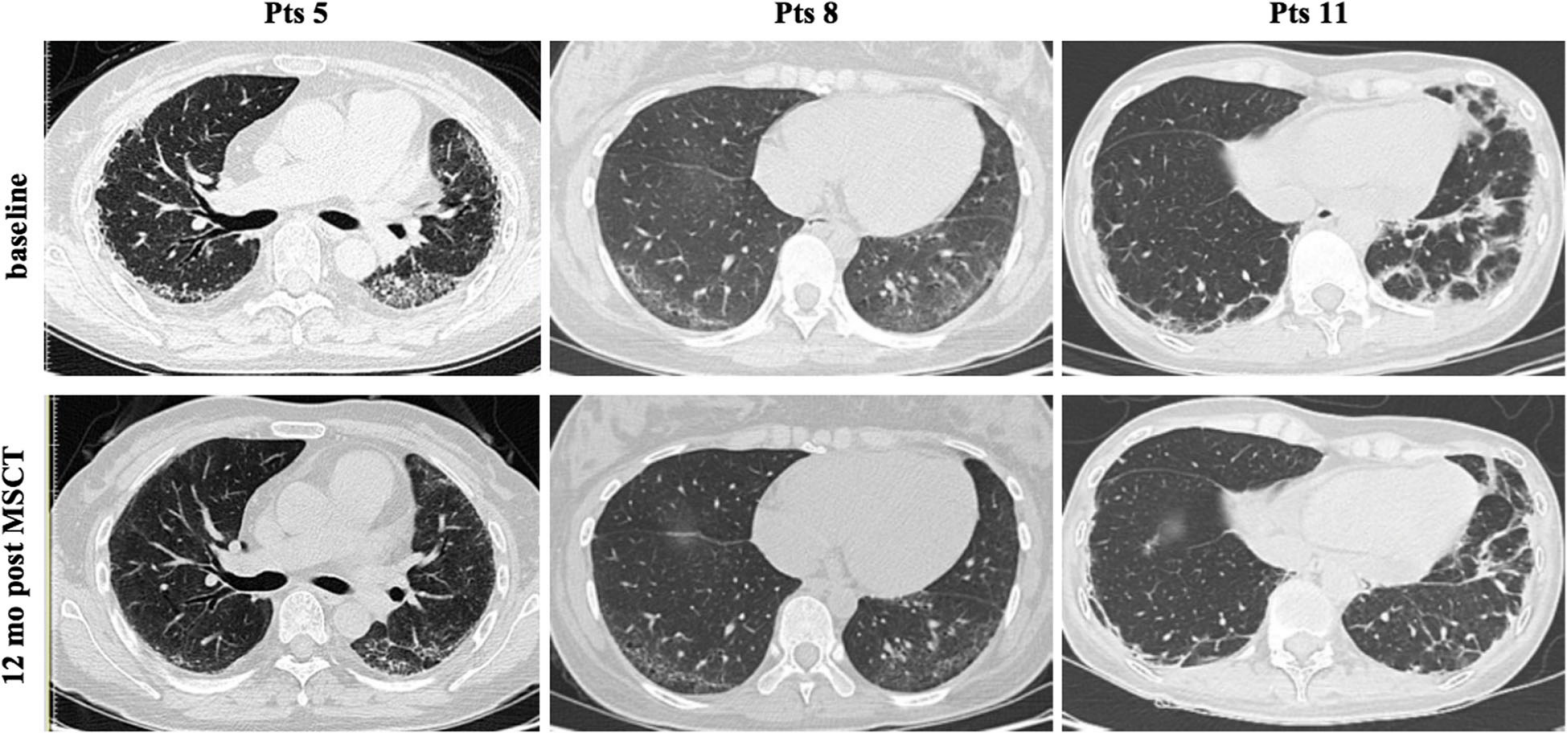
Pulmonary high-resolution computed tomography in patients with systemic sclerosis. *Upper panel* before mesenchymal stem cell transplantation (*MSCT*); *lower panel* 12 months after MSCT. *Pts* patients
